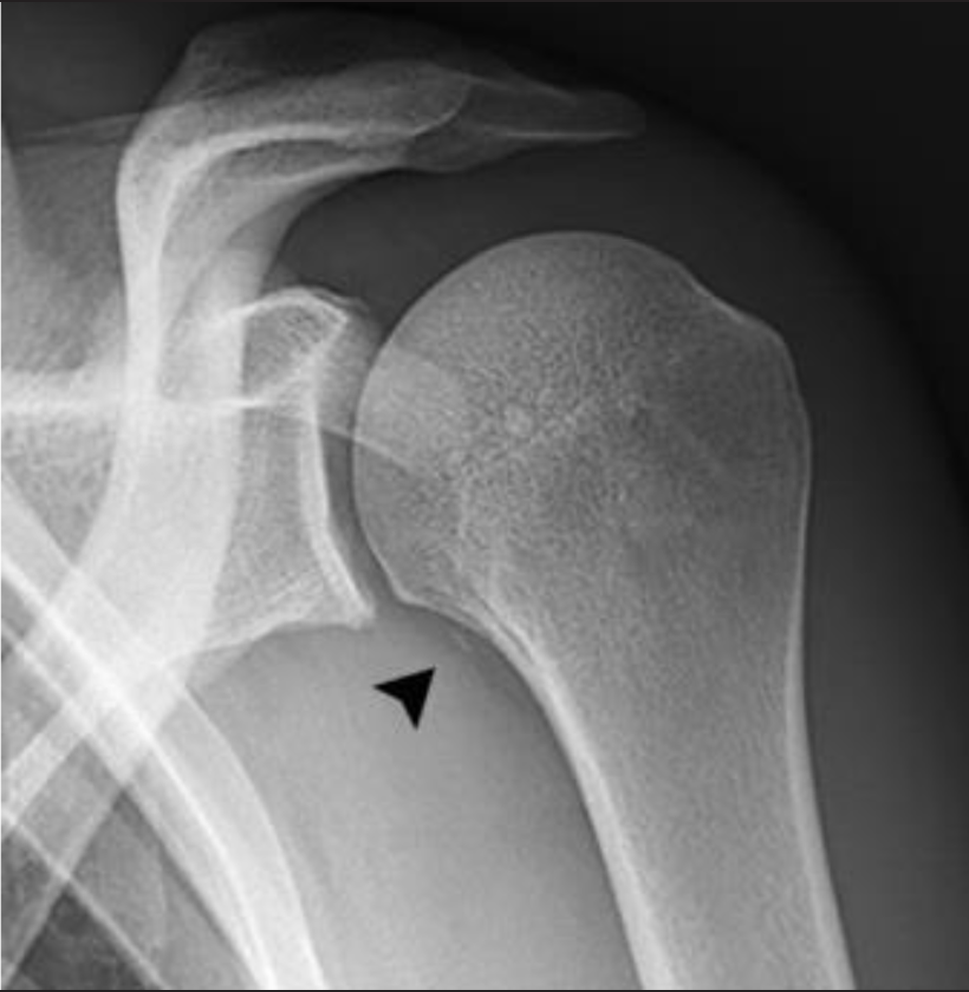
A 29-year-old rugby player presenting after shoulder trauma. Standard radiography of the left shoulder. A small fleck of bone (black arrowhead) is seen medial to the proximal humerus.
